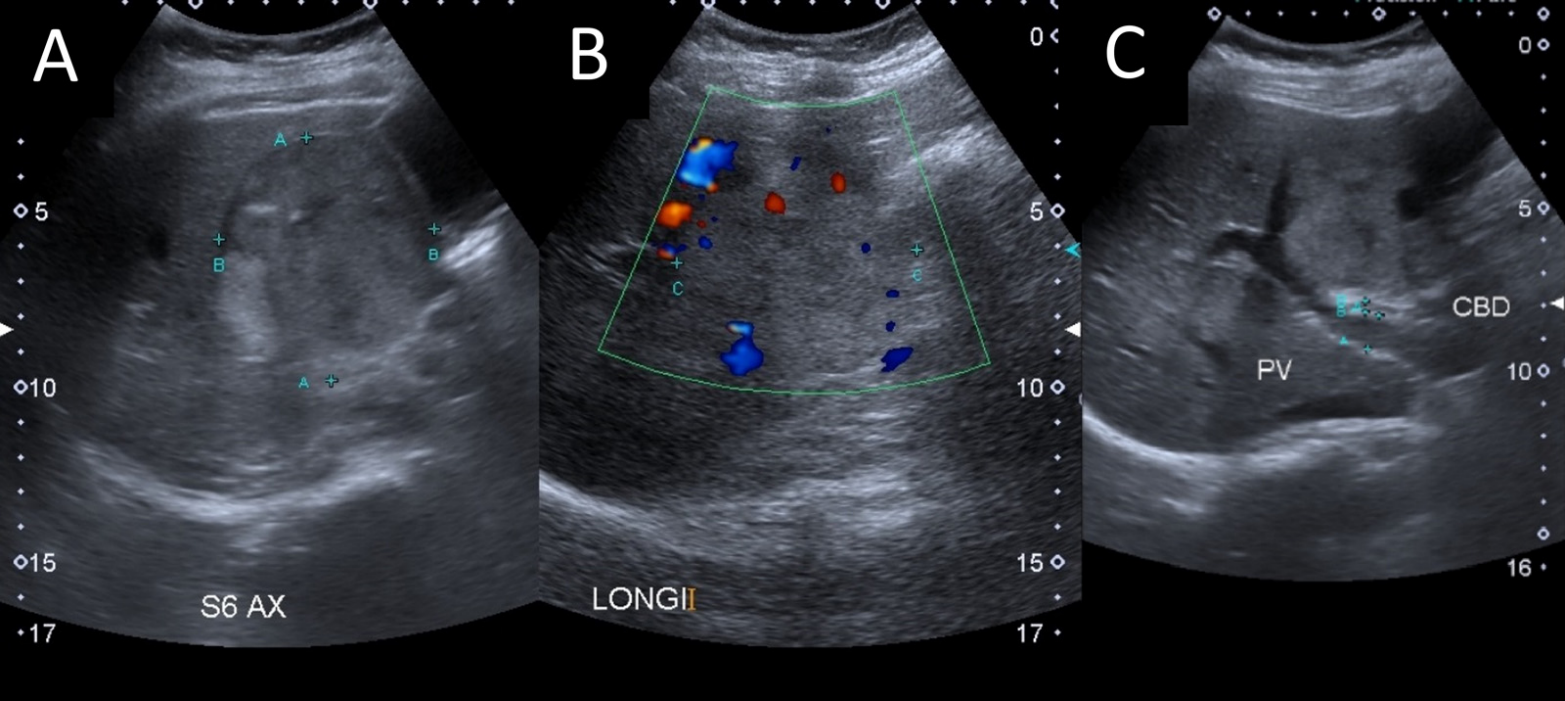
Ultrasound images demonstrating a segment VI liver lesion (A) measuring 6.4 × 6.6 × 7.4cm. The lesion had a focus of calcifications and few cystic lesions. Internal vascularity (B) was seen within. The mass caused effacement of the portal vein and displacement of the gallbladder anteriorly (C).
